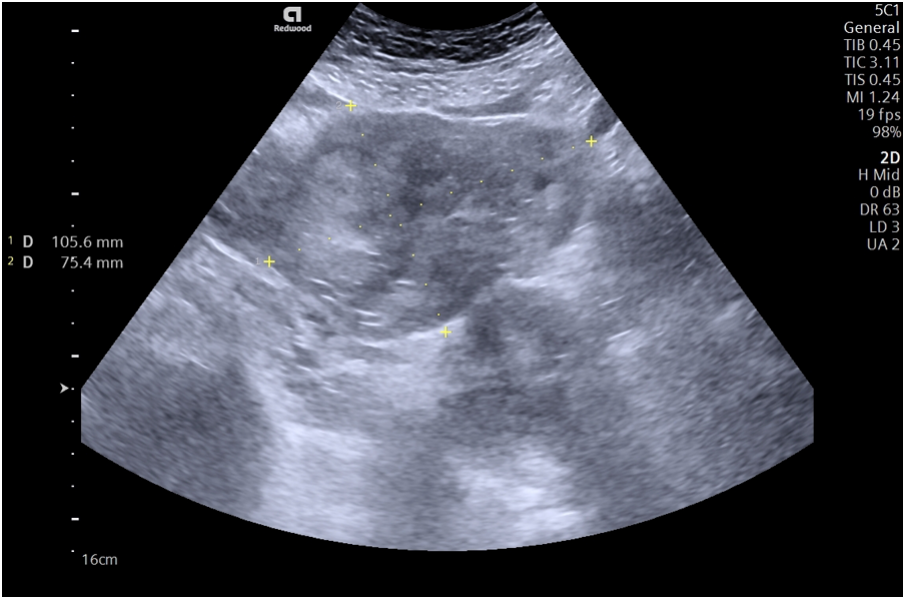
A transvaginal ultrasound showing a formation of heterogeneous mass in the region of the right ovary and fallopian tube.
